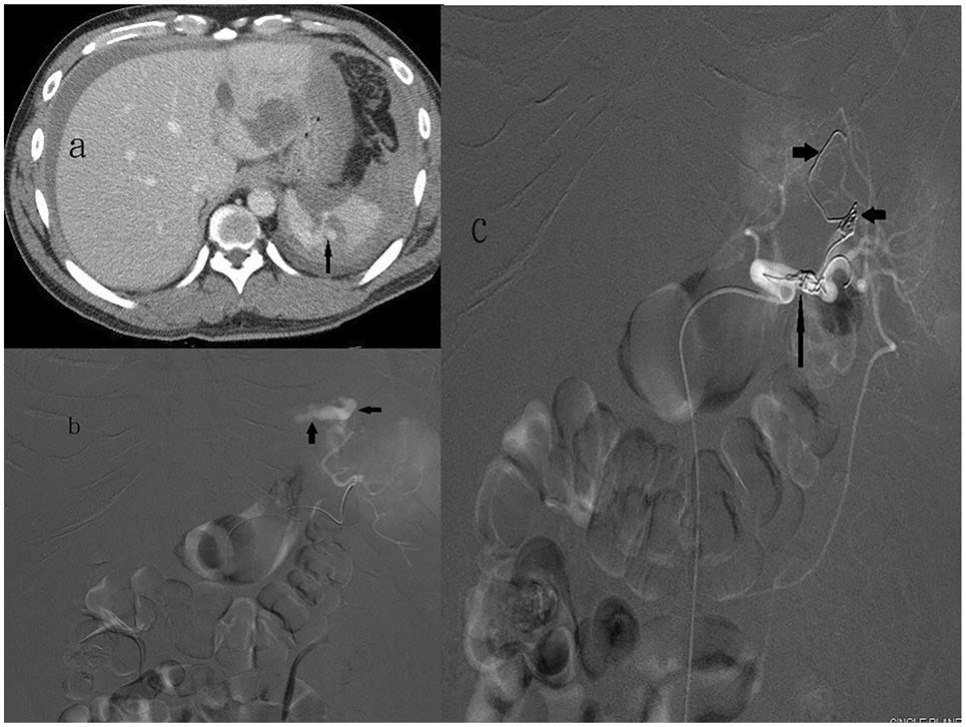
32-Year-old man with a grade IV blunt splenic injury underwent combined splenic arterial embolization. a Admission contrast-enhanced helical computed tomography scan demonstrates a low attenuation laceration with a pseudoaneurysm (arrow) and perisplenic hematoma. b The splenic angiogram demonstrates an active leak of contrast medium from a branch artery of upper pole (arrows). c The post-embolization angiogram demonstrates combined embolization with coils in the proximal splenic artery (arrow) and in the distal branch (arrowheads)
